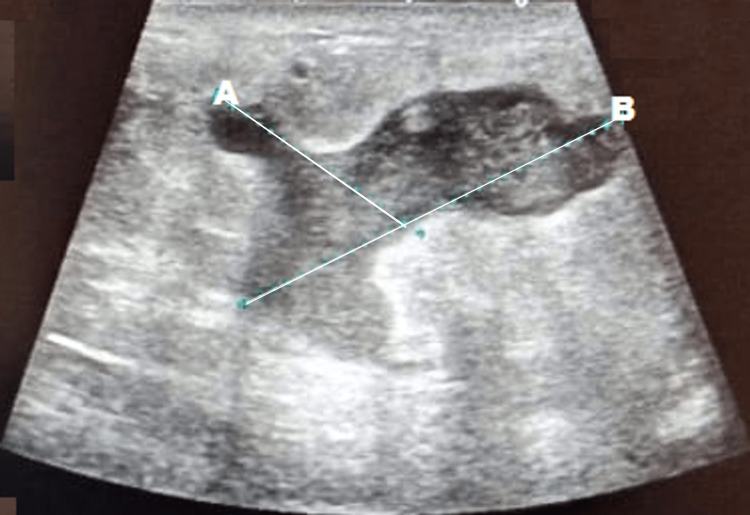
Ultrasonography of the affected breast which demonstrated a lesion in the parenchyma with thin fluid streaks. These findings are marked by the white lines A and B.
